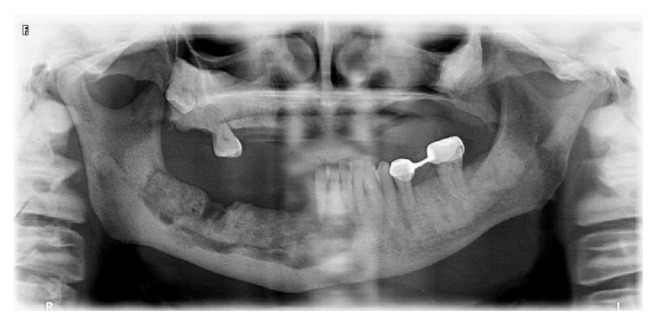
Panoramic radiograph showing osteomyelitis in the right mandibula due to extraction of the right mandibular second molar tooth.
